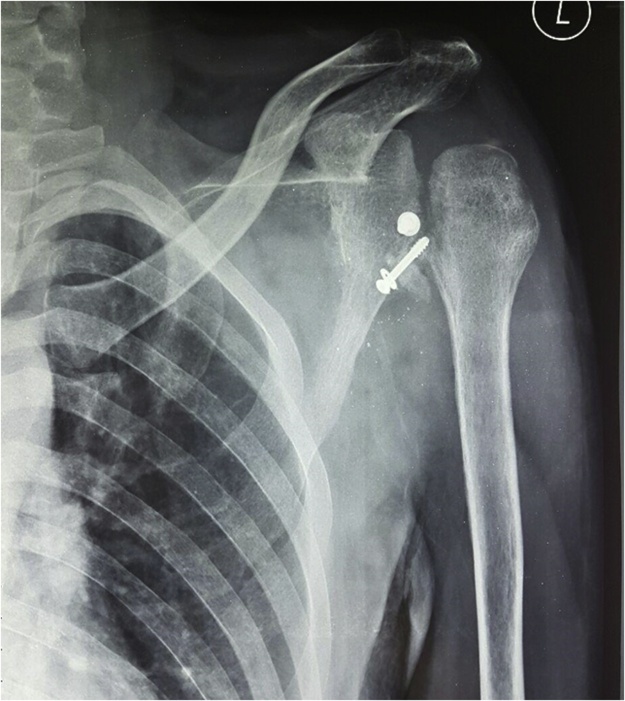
Three months post-operative X-ray antero-posterior view of the left shoulder showing loss of the humeral head contour.
